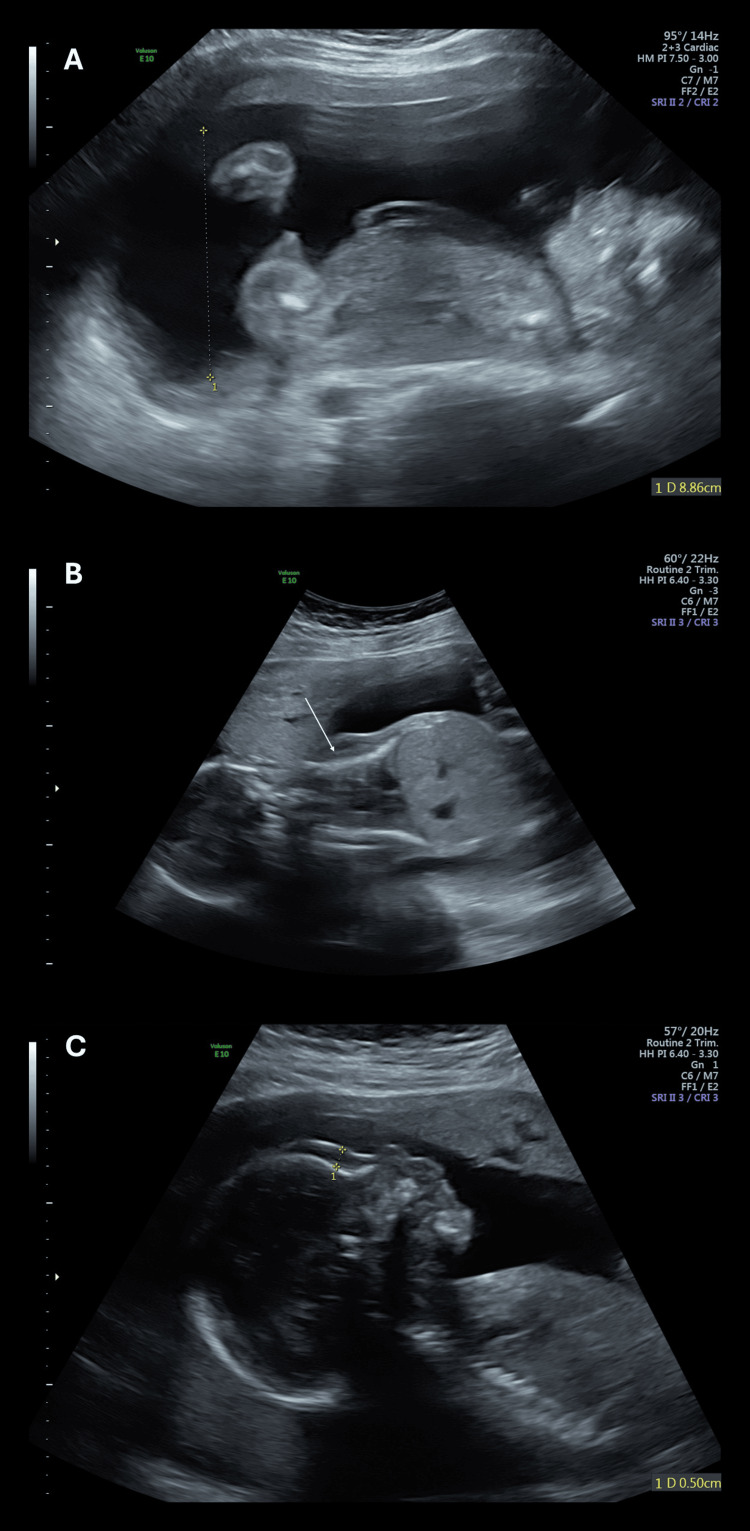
Ultrasound findings suggestive of paternal uniparental isodisomy 14 (A) Maximum vertical pocket (MVP) measurement indicative of polyhydramnios, (B) subjectively narrow chest, (C) abnormal facial profile with thickened skin.
